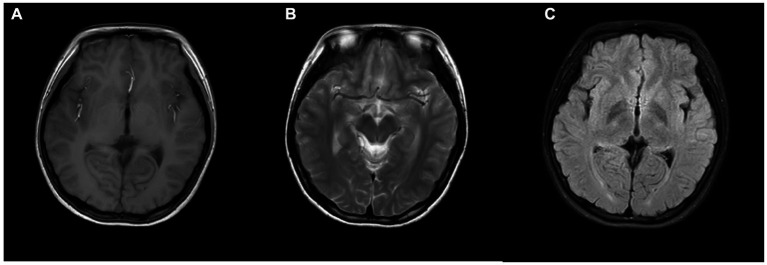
Picture of brain Conventional MRI, (A) T1, (B) T2, (C) FLAIR.
